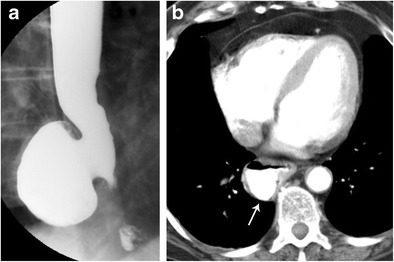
Epiphrenic Diverticulum. a Esophagram in oblique projection demonstrates herniation of the lumen within the lower oesophagus centred less than 10 cm from the gastroesophageal junction consistent with an epiphrenic diverticulum. b CT of the chest with IV and oral contrast in soft tissue window shows contrast filling an outpouching located lateral to the lower thoracic oesophagus
